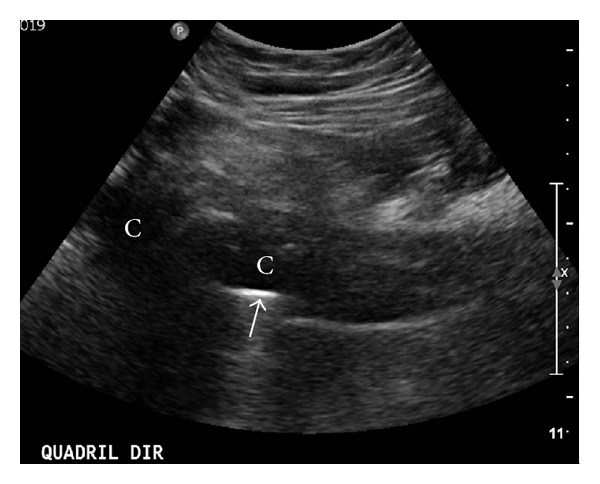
Ultrasound scan on a hip showing thick fluid collections (C) around the femoral component of the hip prosthesis (arrow).
